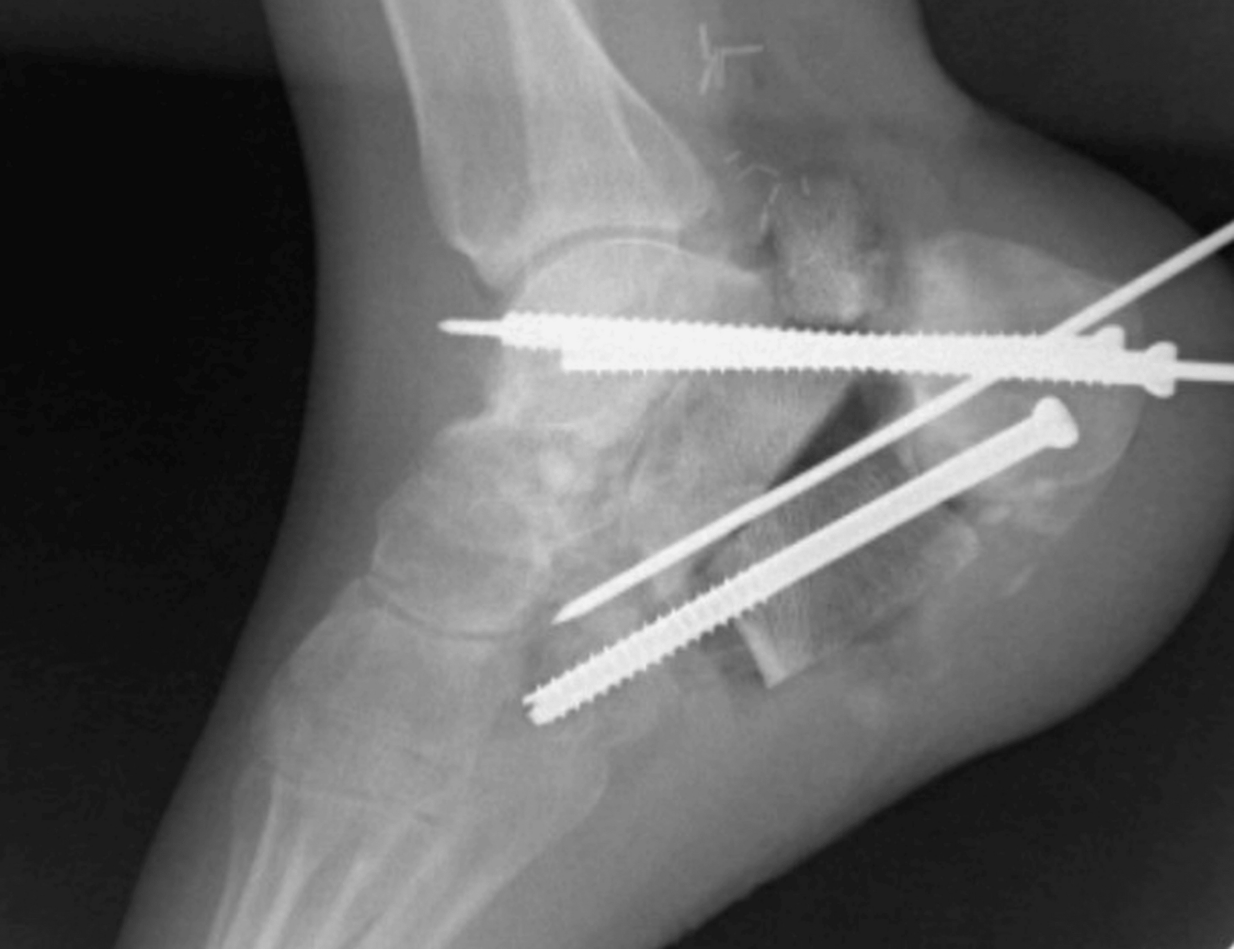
Intraoperative X-ray image showing a large calcaneus bone defect filled with a structural femoral head allograft and arthrodesis of the subtalar and calcaneocuboid joints.
